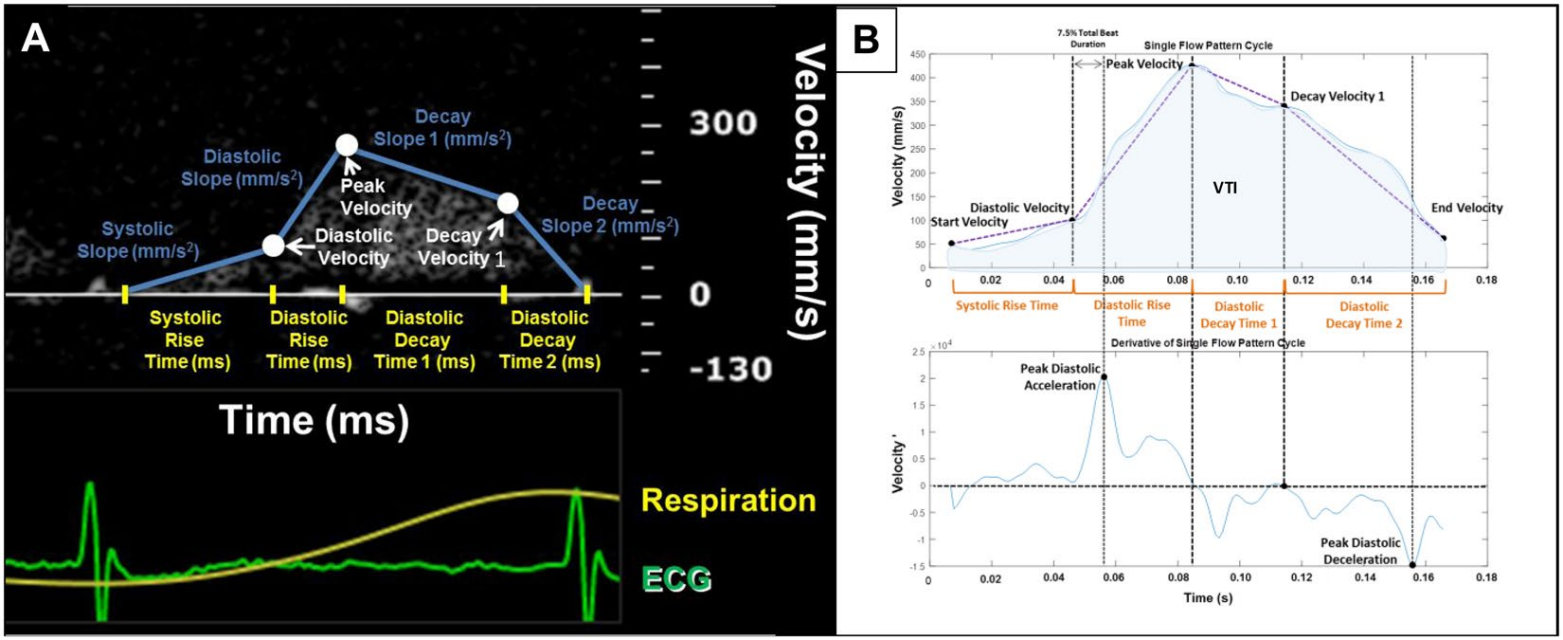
(A) Parameters of coronary flow patterns over the course of one cardiac cycle overlaid on Doppler recording. (B) Example of a single coronary flow pattern cycle and its corresponding derivative. The velocity and acceleration points (black) were first identified. From there, the time intervals were measured (orange) and the average slopes were calculated (purple).
